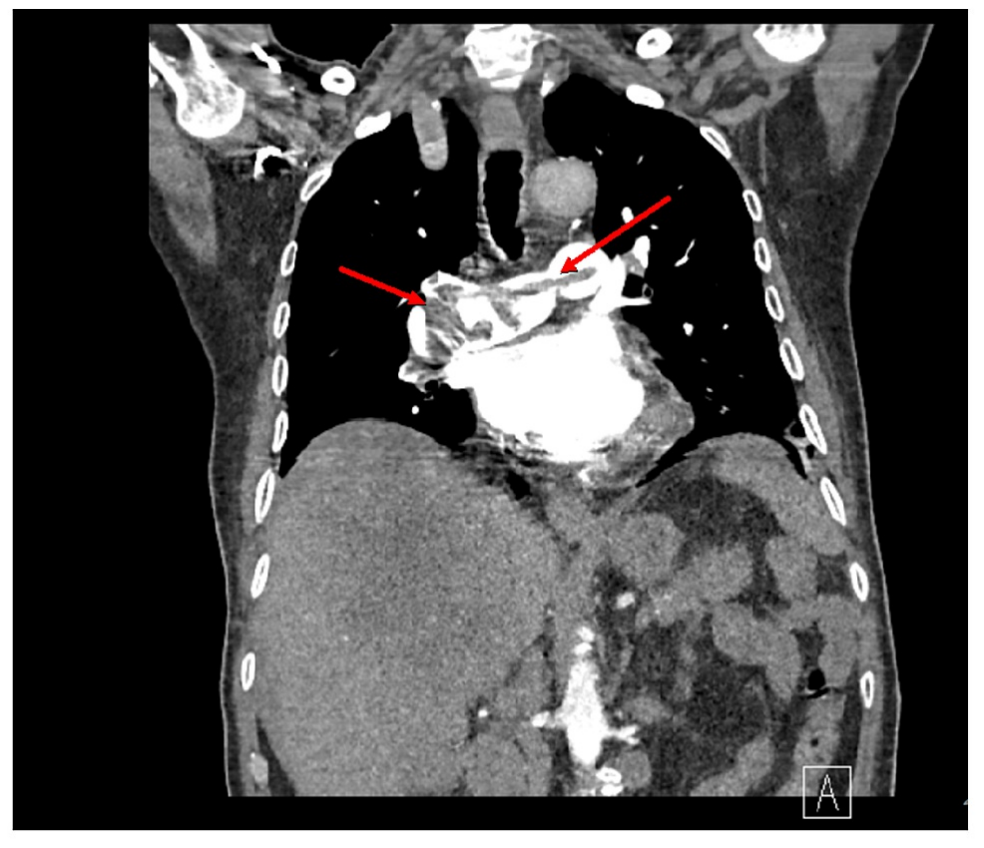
Axial CT scan of the chest for confirmation of the saddle pulmonary embolism
